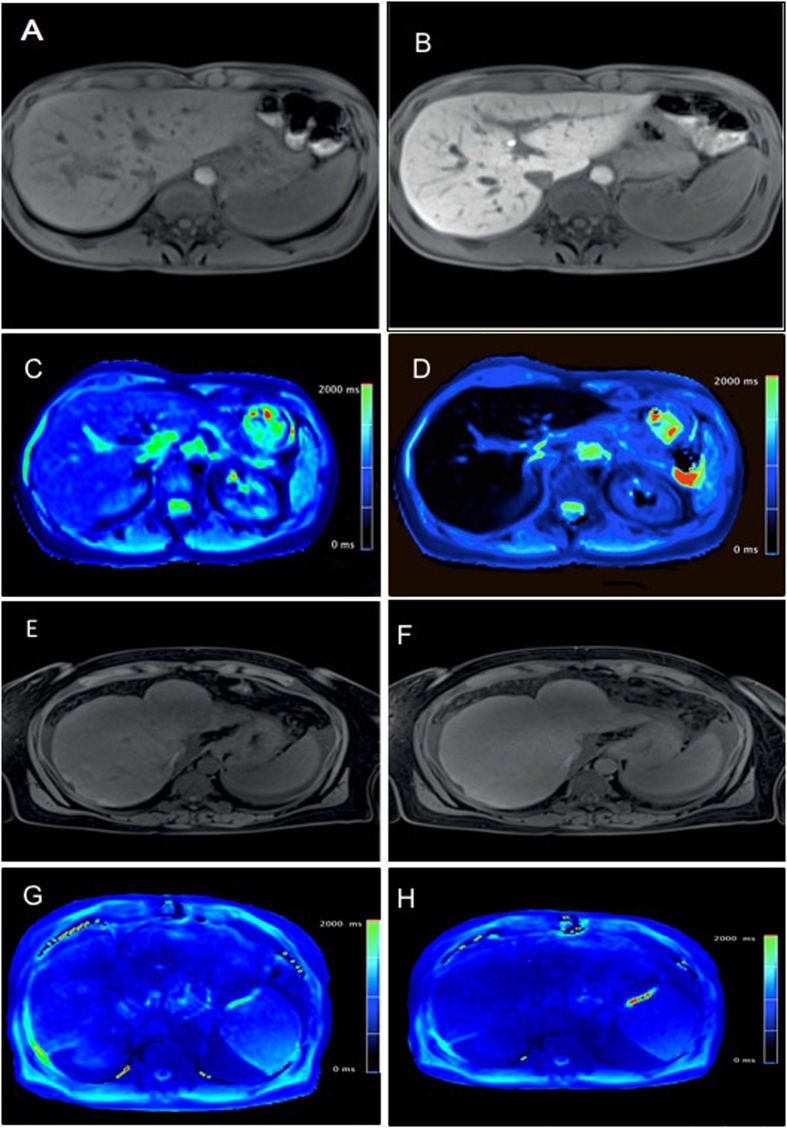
T1-weighted VIBE sequences and colour-coded T1 maps for patient with normal and impaired liver function. T1 weighted VIBE sequences (A,B,E,F) and T1 maps (C,D,G,H) with inline correction for B1 heterogeneities were obtained before and 20 min after the administration of Gd-EOB-DTPA in a 45-year-old patient with normal liver function (A–D; ICG-PDR = 29.1%/min) and in a 62-year-old patient with impaired liver function (E–H; ICG-PDR = 3.6%/min).
